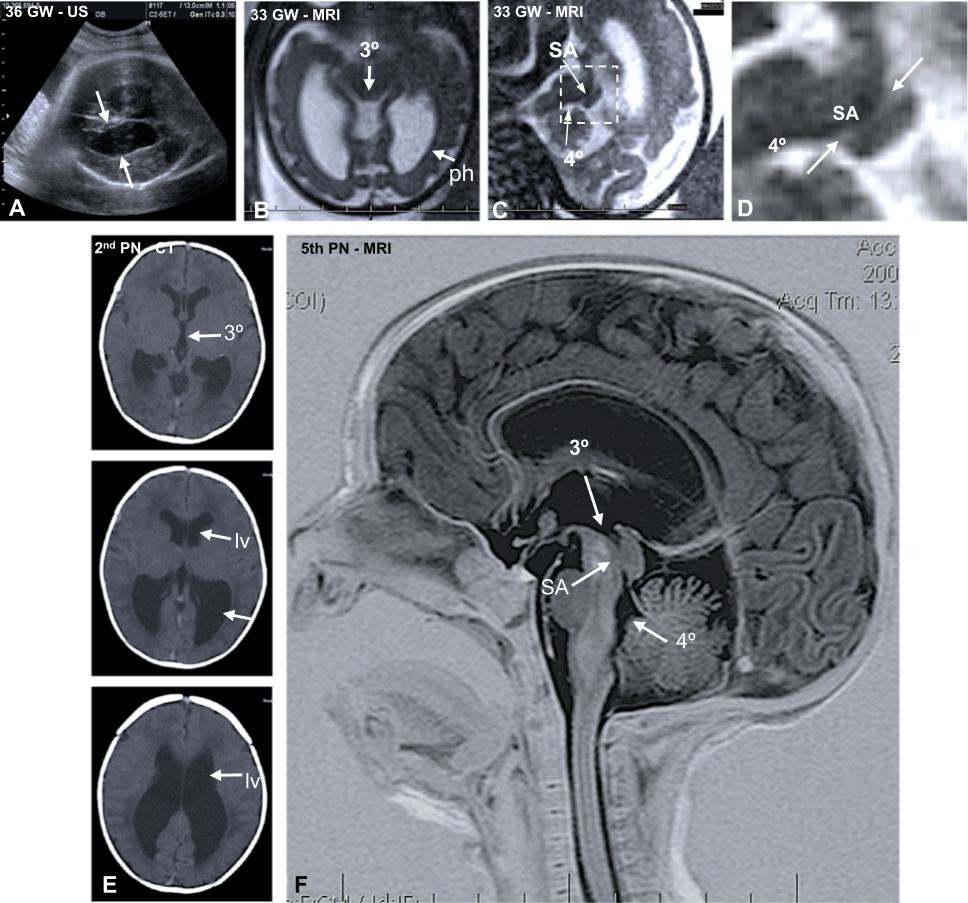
Progressive obliteration of the aqueduct of Sylvius (SA) in hydrocephalus. a An ultrasound of the fetal patient at 36 GW demonstrating dilation of the lateral ventricles (arrows). b MRI at 33 GW. The third ventricle (3°) and posterior horns of the lateral ventricles (ph) are dilated. c MRI at 33 weeks. Stenosis of the SA is shown. d Detailed magnification of the area framed in C showing stenosis of the SA. 4°, fourth ventricle. e CT at 39 GW (or the 2nd PN day). The lateral (LV) and third (3°) ventricles are dilated. f MRI of the brain on the 5th postnatal day, sagittal T2 imaging. The SA is obliterated
